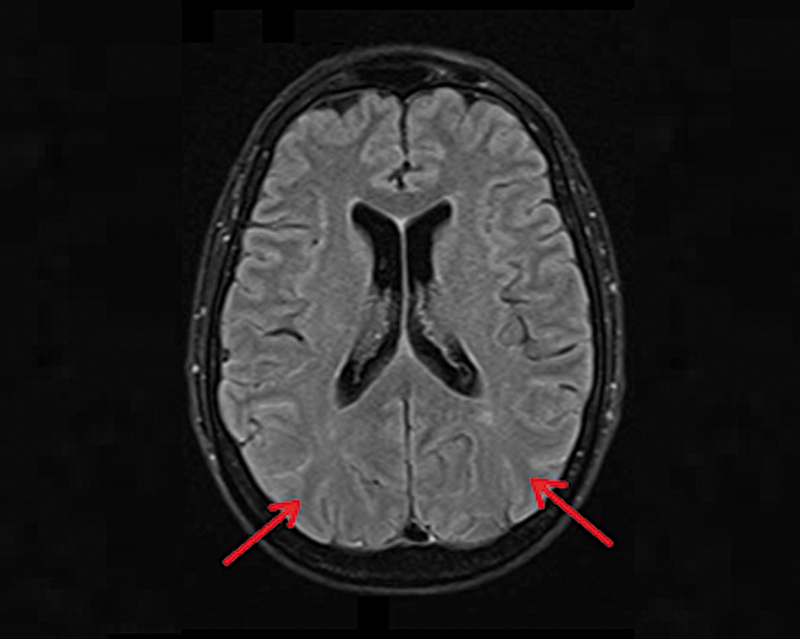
Magnetic resonance imaging brain T2-FLAIR sequence. Areas with a mild loss of signal saturation of cerebrospinal fluid in certain cortical sulci of both cerebral hemispheres.
